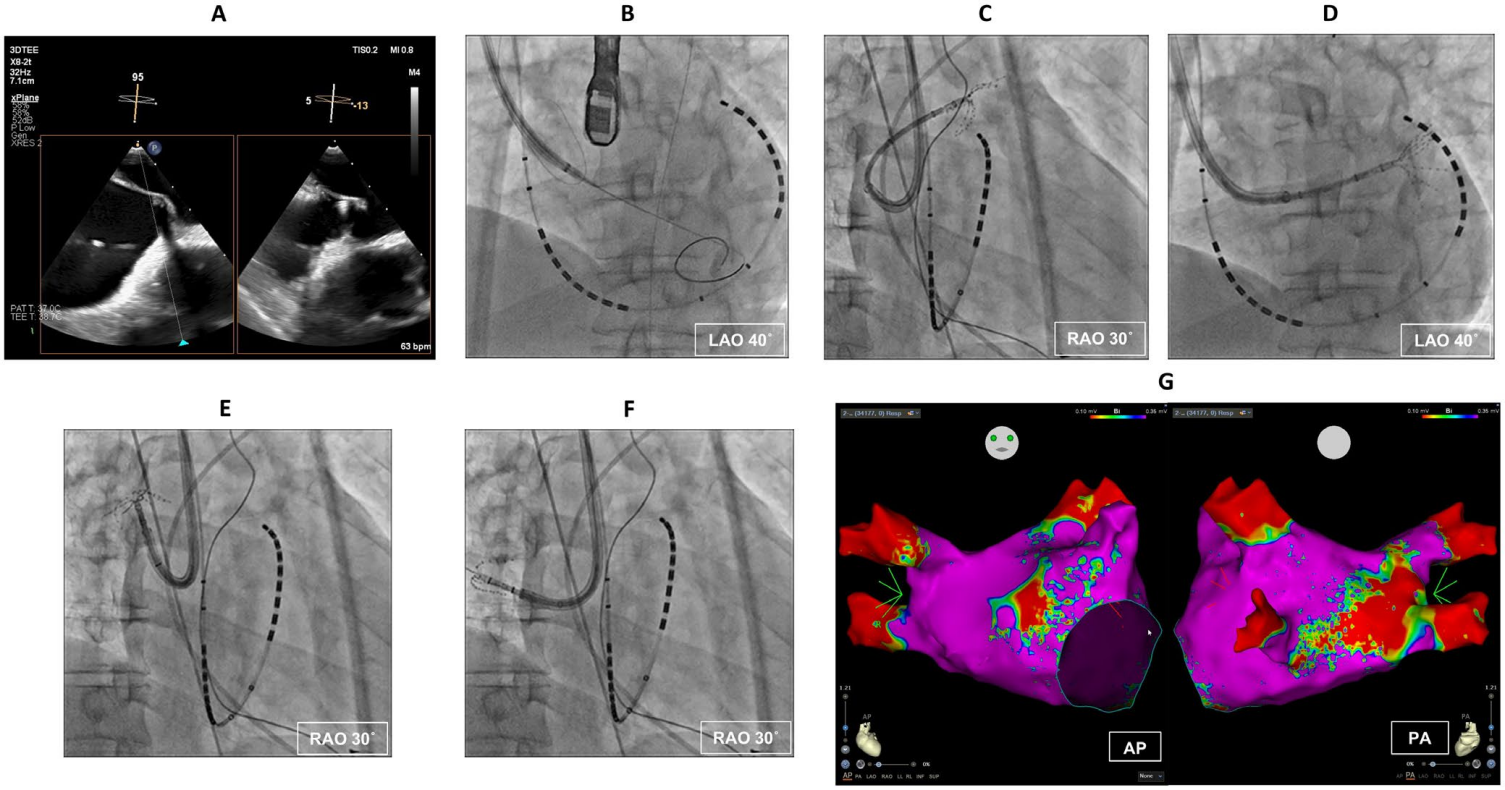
Transseptal puncture and pre‐ablation left atrial voltage mapping. (A) Transesophageal echocardiographic image at the transseptal puncture. (B) Insertion of a radiofrequency wire to left ventricle. (C–F) Voltage mapping of left superior, left inferior, right superior, and right inferior pulmonary veins using a multielectrode mapping catheter (OctaRay, Biosense Webster, Irvine, CA) during atrial fibrillation. (G) Left atrial volage map before ablation. Red and purple colors on the voltage map indicate volages of ≤ 0.1 mV and > 0.35 mV, respectively. PA, posteroanterior; RAO, right anterior oblique; the other abbreviation is defined as in Figure 1.
